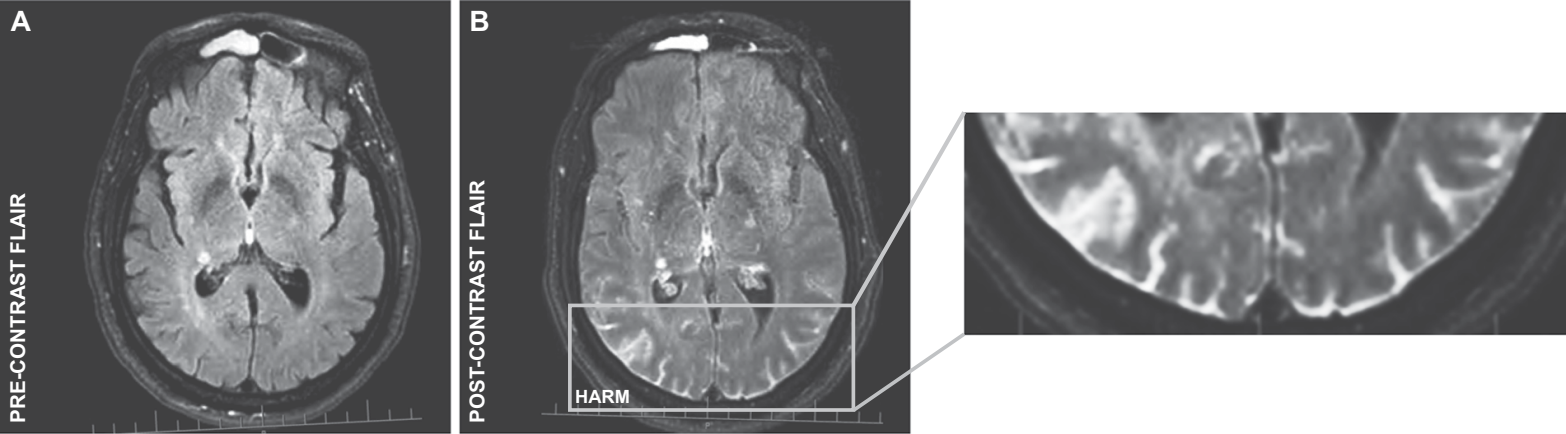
Assessment of post-stroke BBB disruption via HARM on perfusion-weighted imaging. (A) Pre-contrast and (B) post-contrast FLAIR images from a patient presenting with HARM at 24 hour follow up. HARM was positively identified when the CSF space in the sulci or ventricles appeared hyperintense post-contrast. Level of HARM was systematically categorized as none, mild, moderate, or severe based on the number of serial slices exhibiting evidence of HARM.
